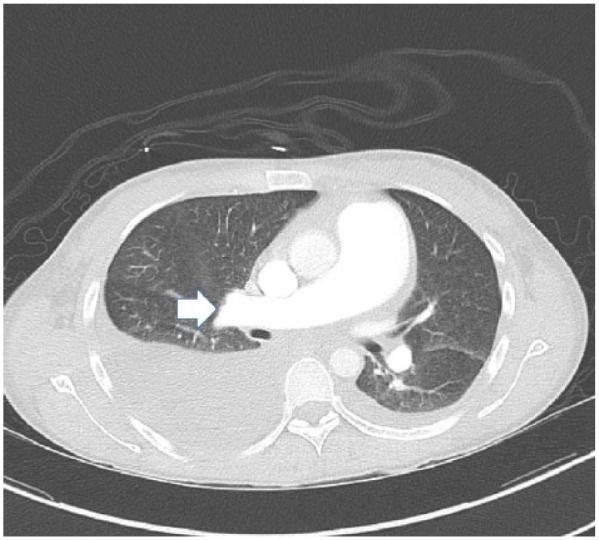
Computed tomography with contrast, axial cut showing markedly dilated pulmonary trunk (arrow).
